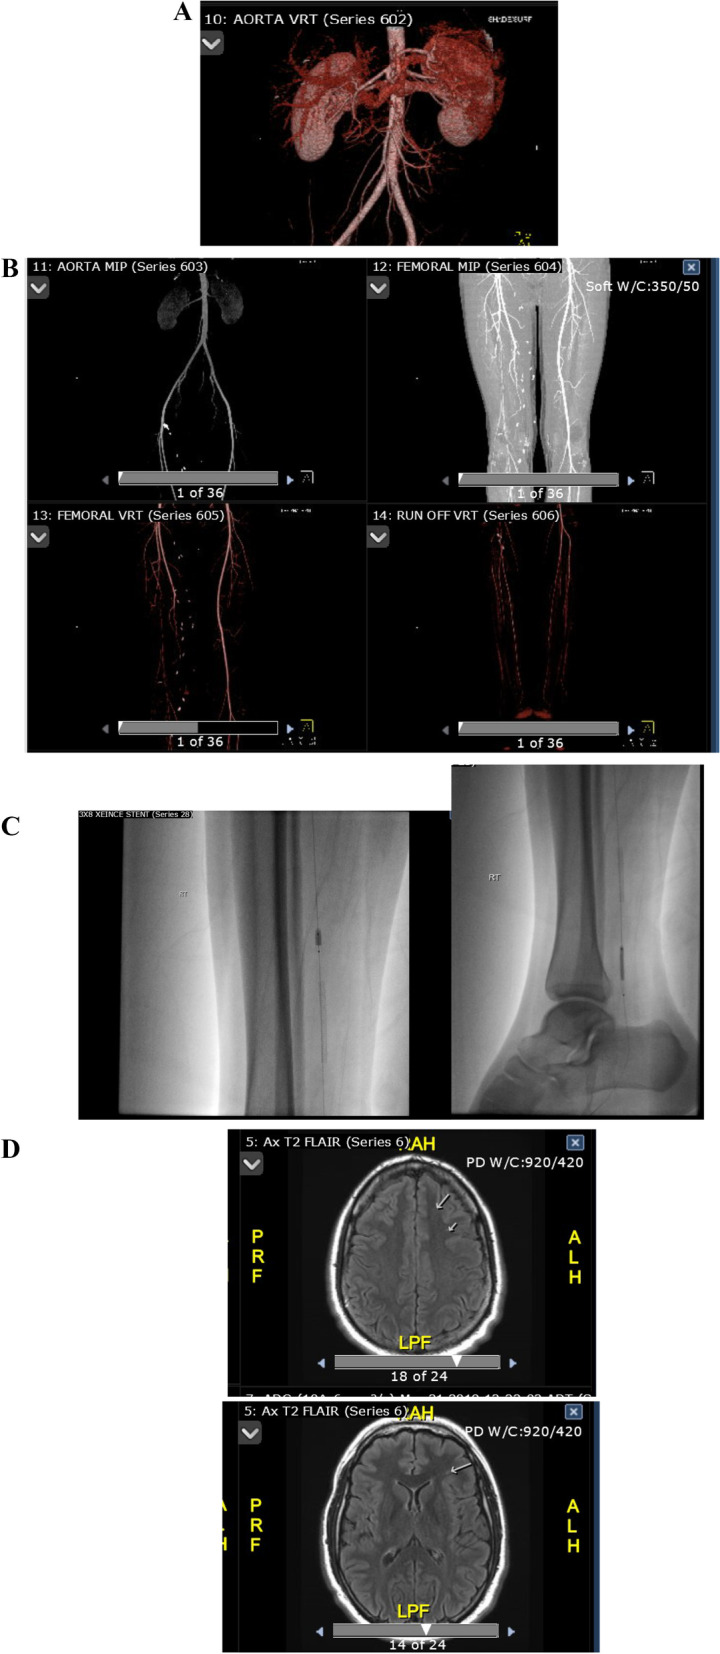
A Abdominal CT Scan Demonstrating Patent Abdominal Aorta. B Patent aortic bifurcation but right superficial femoral artery (SFA) was occluded along with the venous graft connecting the distal SFA to the tibioperoneal trunk. C Successful subintimal recanalization and angioplasty was performed of the right distal posterior tibial artery occlusion as well as the proximal peroneal artery occlusion. D MRI brain scan demonstrating a few tiny left frontal subcortical and periventricular T2/FLAIR hyperintense nonspecific foci
